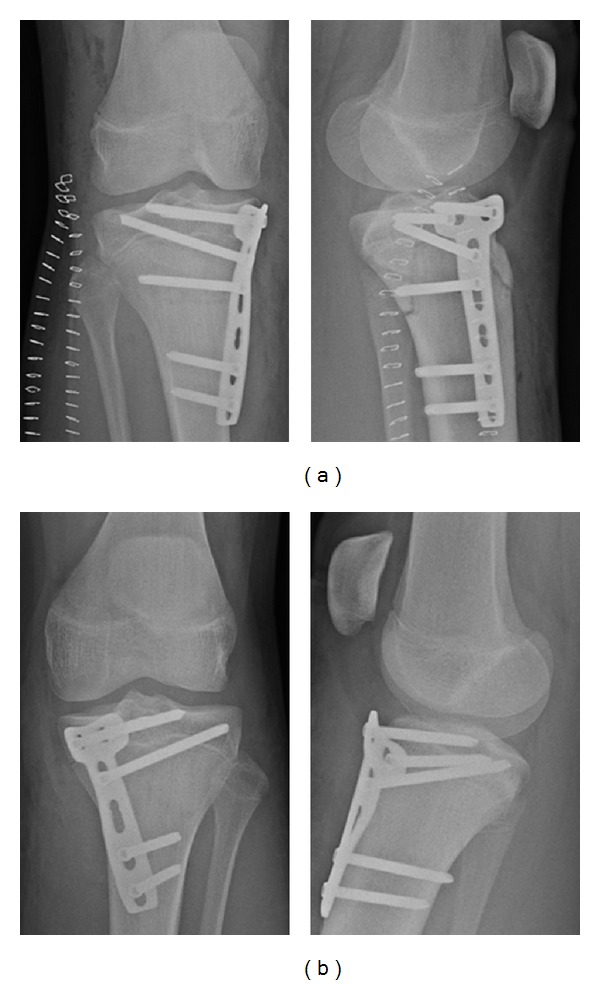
Plain radiographies of the right (a) and left knee (b) after closed reduction and MIPO using two 4.5 mm LCP t-plates placed at the anteromedial aspect of the tibia. Growth plates were bridged.
